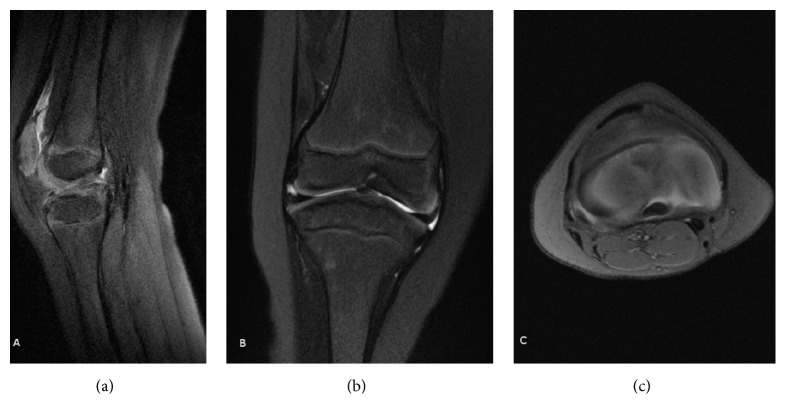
Preoperative MRI (sagittal (a), coronal (b), and transverse (c) views) of the right knee of the patient from Figures 1 and 2 showing the same findings as those in the left knee.
